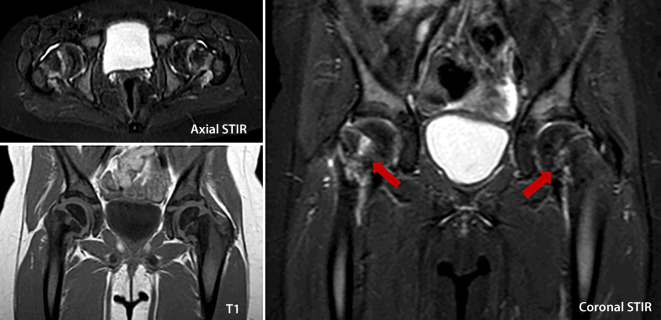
Preoperative MR imaging of the pelvis: STIR sequence axial and coronary shows clear bone edema in both femoral necks (Red arrows) with a T1 correlate, compatible with bilateral femoral neck fracture and slight hip joint effusion. *STIR* Short tau inversion recovery
